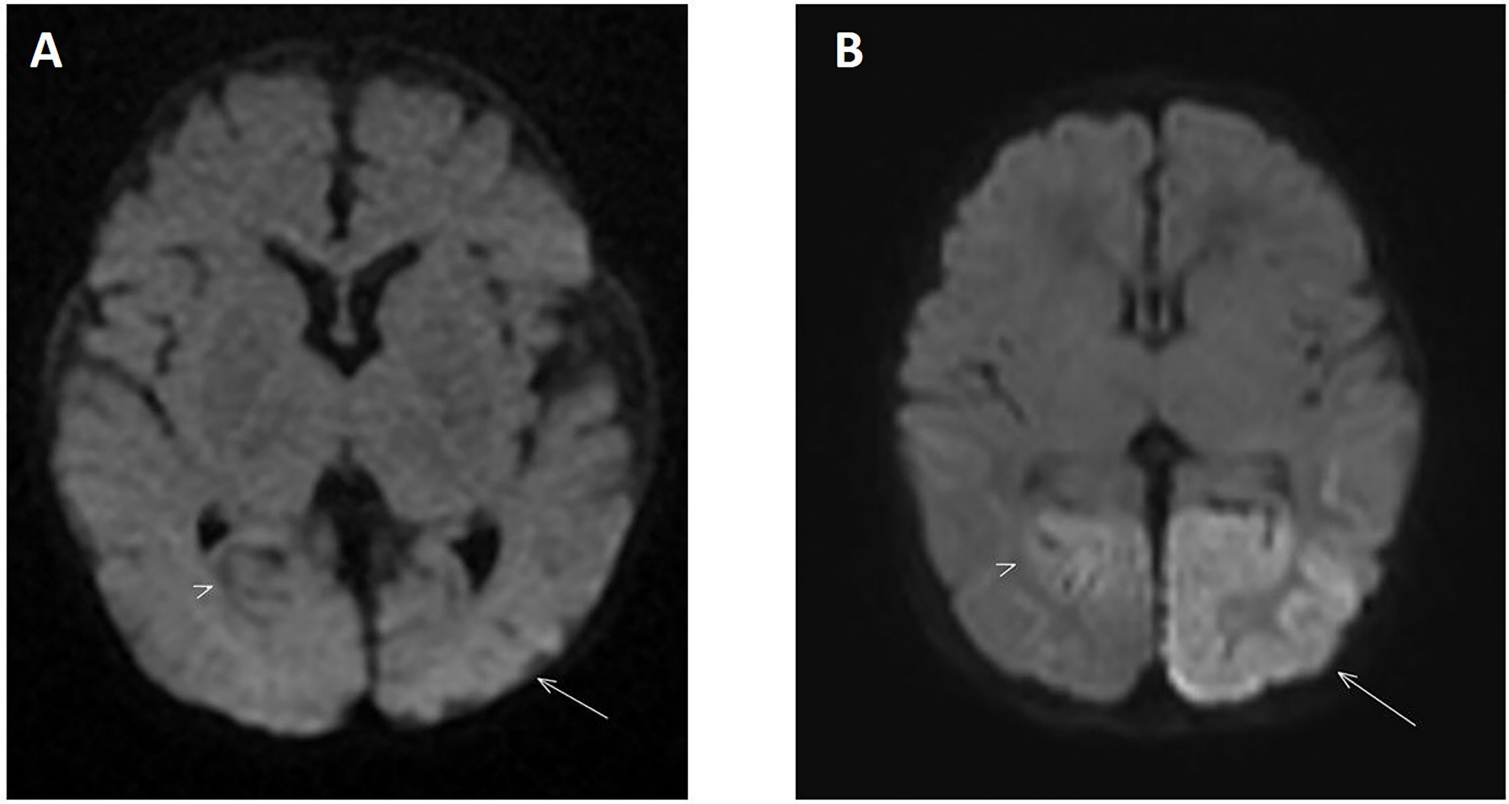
(A) Diffusion-weighted MRI on day 4 of life showing increased signal intensity located in the left occipital lobe (arrow) consistent with an area of restricted diffusion. The right occipital lobe is also involved to a lesser extent (arrowhead). These findings are consistent with severe symptomatic hypoglycemia when correlated to clinical and laboratory investigations. (B) Diffusion-weighted MRI performed 2 months later shows resolution of the restricted diffusion in the previously involved areas. However, there is now residual atrophy in both occipital lobes with the left side more severely involved compared to the right (arrow and arrowhead respectively).
